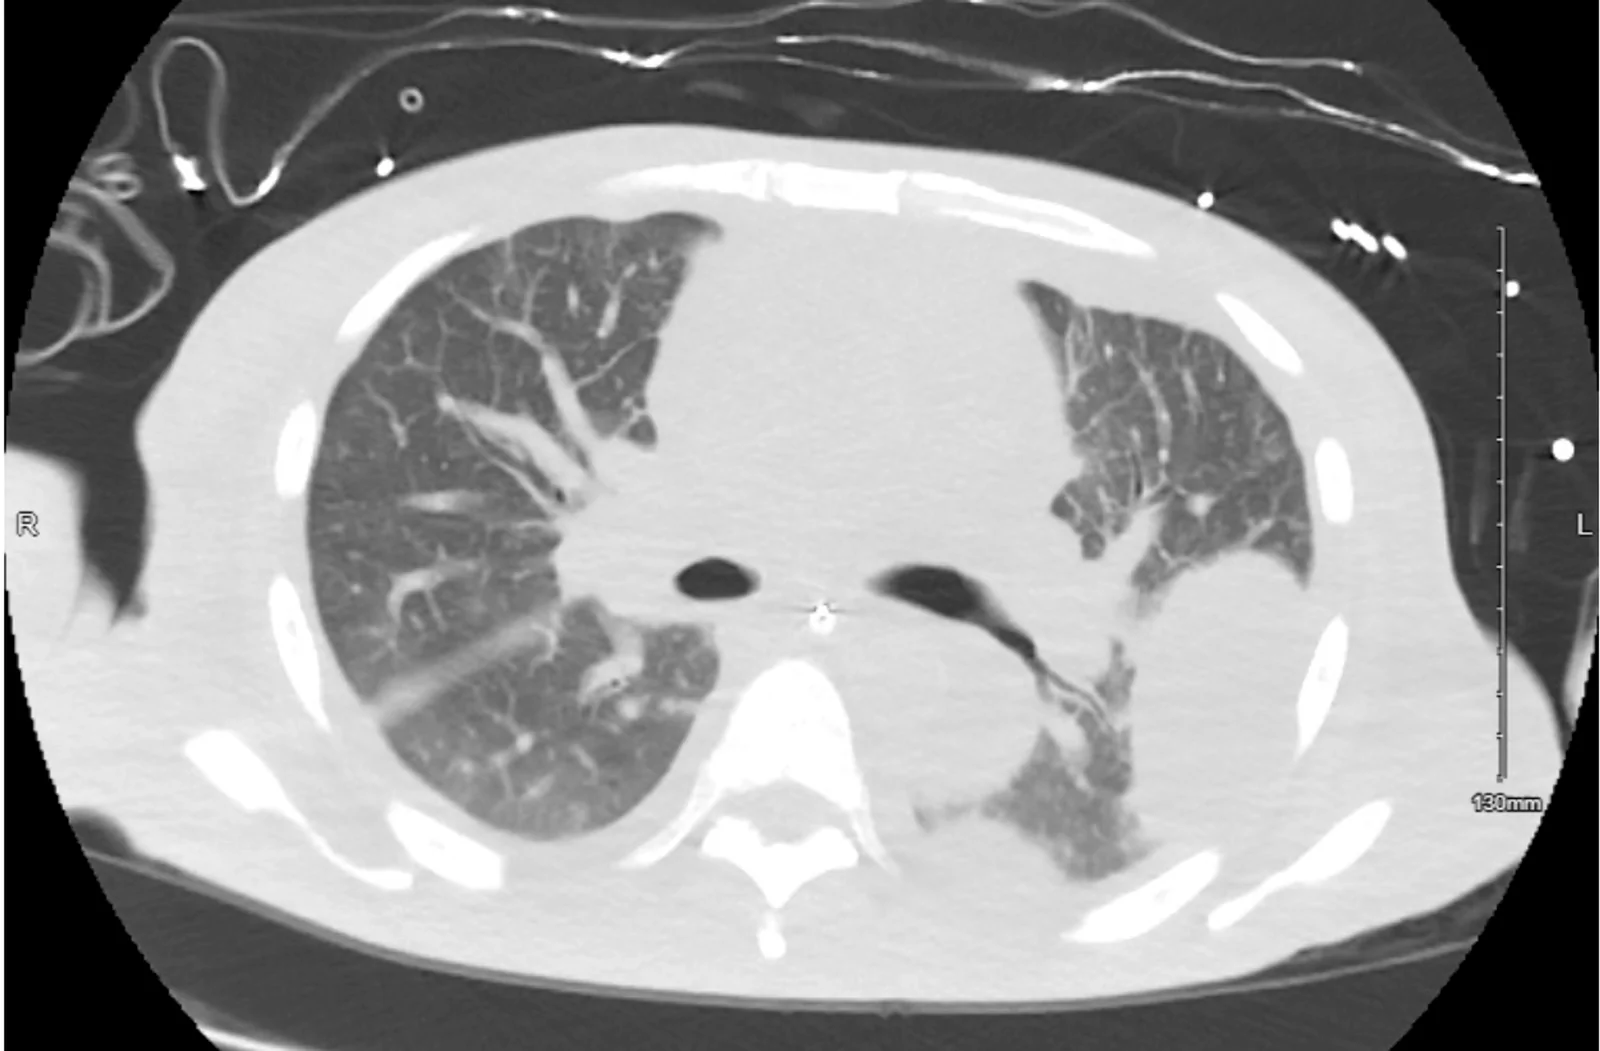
Non-contrast CT Chest and Abdomen Demonstrating Bilateral Loculated Pleural Effusions Computed tomography imaging demonstrating worsening bilateral loculated pleural effusions with largest component in the left pleural space, in the setting of advanced pseudomyxoma peritonei.
